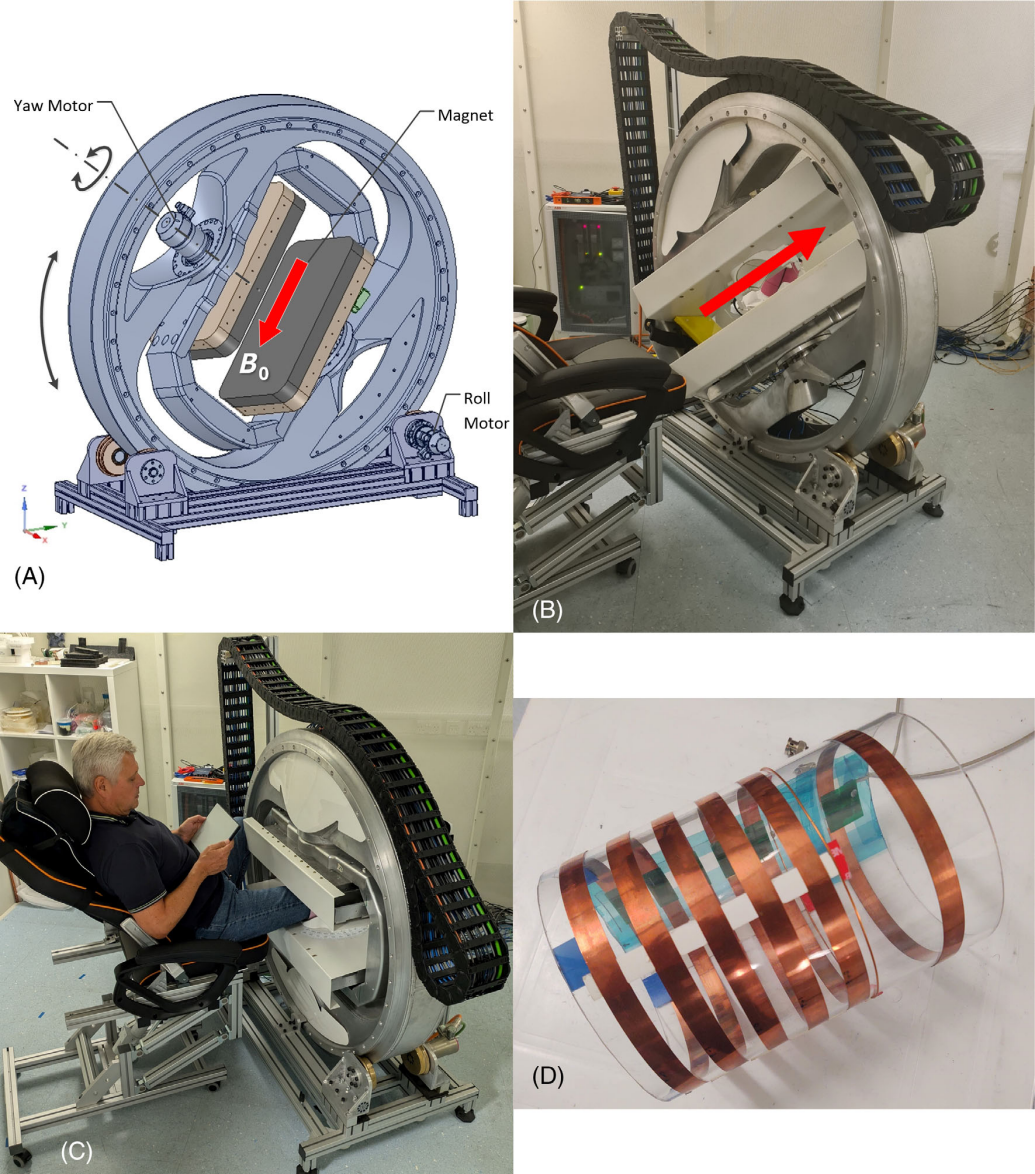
MA MRI, dedicated to extremity imaging, employing low‐field, transverse field open magnet and rotation about two orthogonal axes (*roll* and *yaw*). (A) CAD model showing the magnet arrangement and 2 degrees‐of‐freedom actuation. (B) Magnet in an arbitrary position with $B_{0}$ direction indicated. (C) Magnet and volunteer during scanning. (D) Construction of the solenoid receiver consisting of five inductively coupled resonator loops. The resonator is tuned to 6.4 MHz as the frequency of the first resonant mode, and it is inductively coupled to the preamplifier and detuning circuitry.
